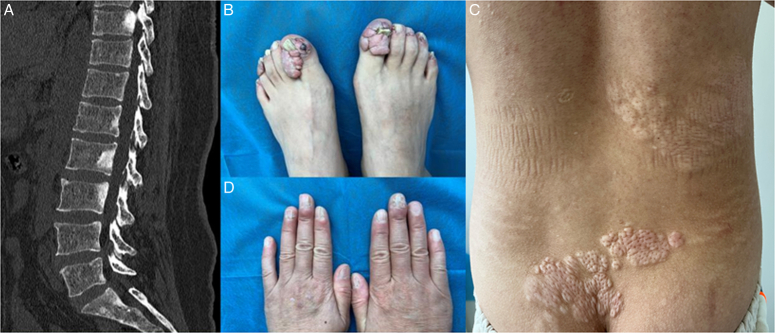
(A) Lumbar sagittal computed tomography reconstruction for a middle-aged woman with tuberous sclerosis complex revealed multiple sclerotic lesions in the middle and posterior vertebral columns. (B, C) Physical examination showed multiple fleshy nodules under fingernails and toenails (ungual fibromas). (D) Her young son had some thick, leathery, pebbly skin abnormalities (Shagreen patches) on the back.
